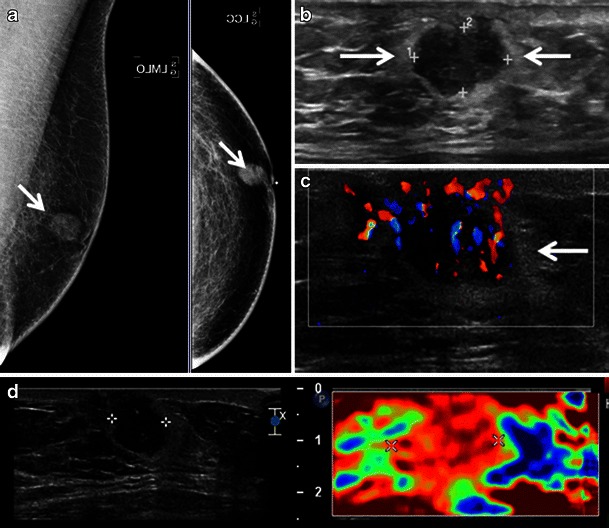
A 73-year-old man with a history of CLL and a new palpable mass in the left breast with associated nipple retraction. Biopsy showed chronic lymphocytic leukaemia. a Left breast medio-lateral oblique and cranio-caudal mammograms show an oval circumscribed subareolar mass with mild nipple retraction (*arrows*). b, c Ultrasound demonstrates an irregularly shaped, hypoechoic mass with angular margins and a thick echogenic rim which shows increased vascularity on colour Doppler evaluation (*arrows*). d Strain elastography (*red* equating to hard) demonstrates a hard mass which is significantly larger on the elastogram. The ratio of size was 1.99 between the B-mode and strain image.
